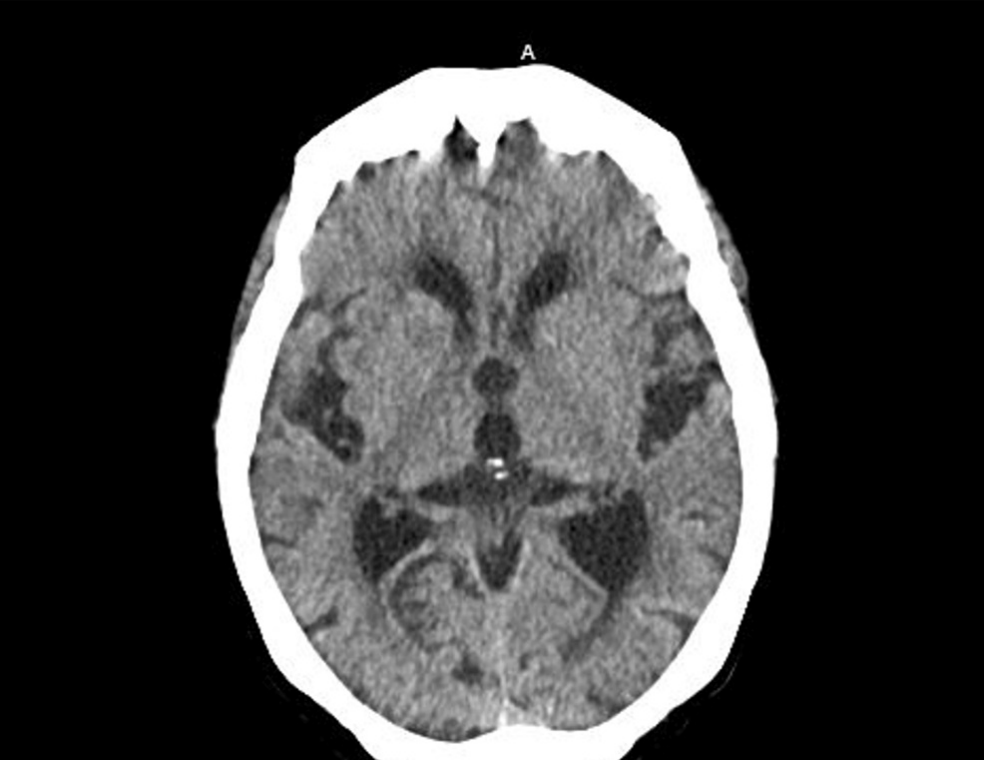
Computed tomography on admission showing no acute intracranial process with progression of diffuse cerebral atrophy, chronic microangiopathic ischemic changes, and an old lacunar infarct in the right basal ganglia
